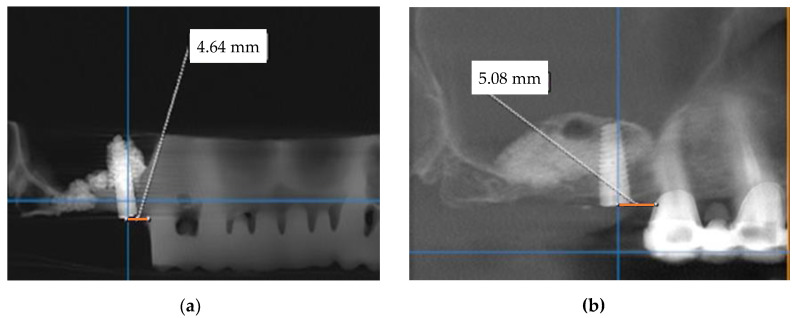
Measurement of the mezio-distal position of the implant (i.e., distance from the anterior tooth to the middle of the implant (D1)) on (a) the training CBCT model (D1-3D) and on (b) the postoperative CBCT (D1-P)—panoramic view.
